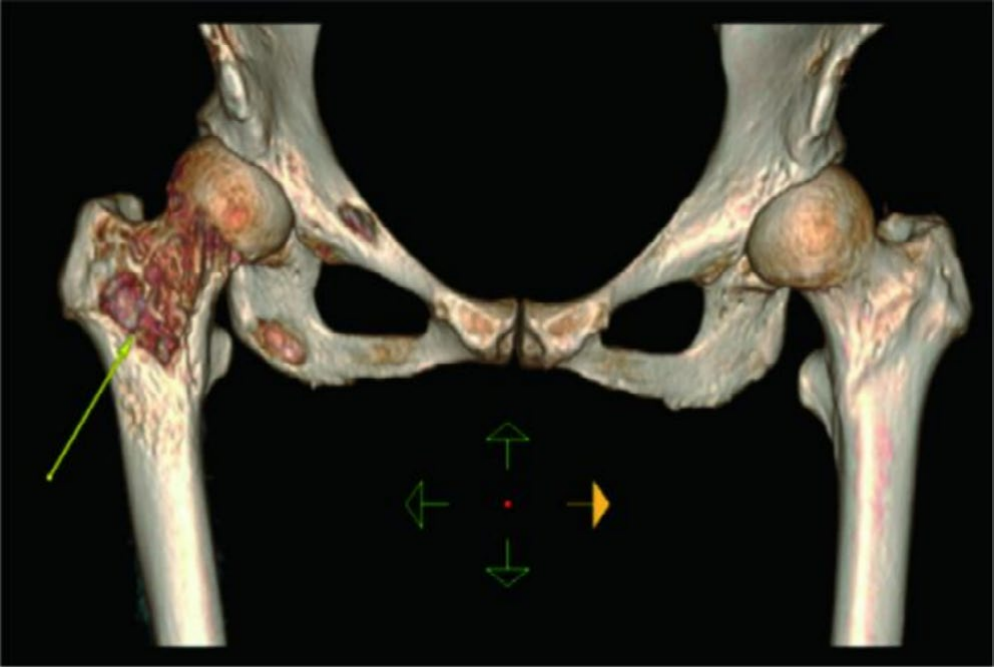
CT reconstruction of right femur metastasis.
